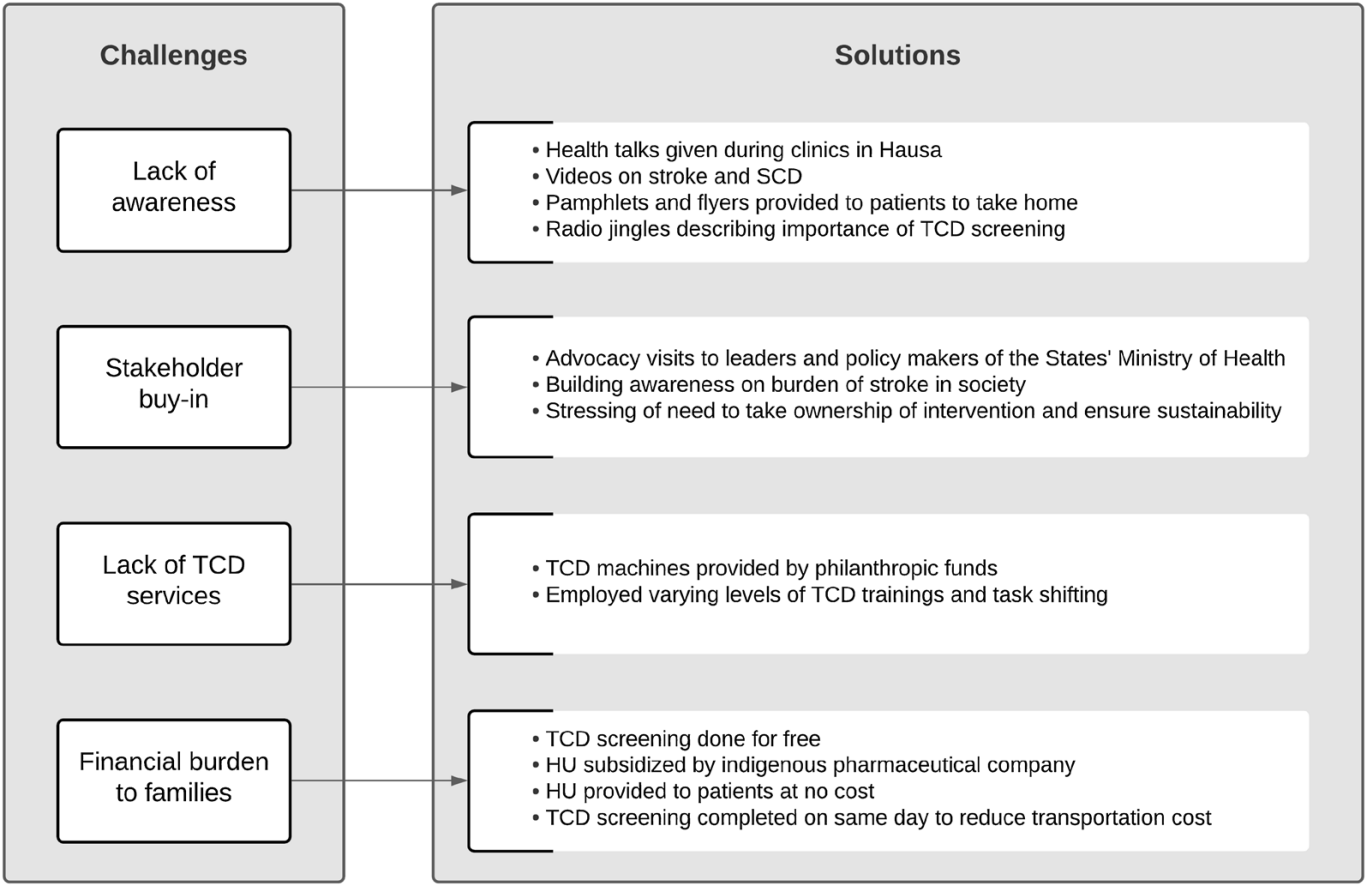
Challenges to establishing a stroke prevention program in northern Nigeria and how they were addressed. (*HU* hydroxyurea, *TCD* Transcranial Doppler)
